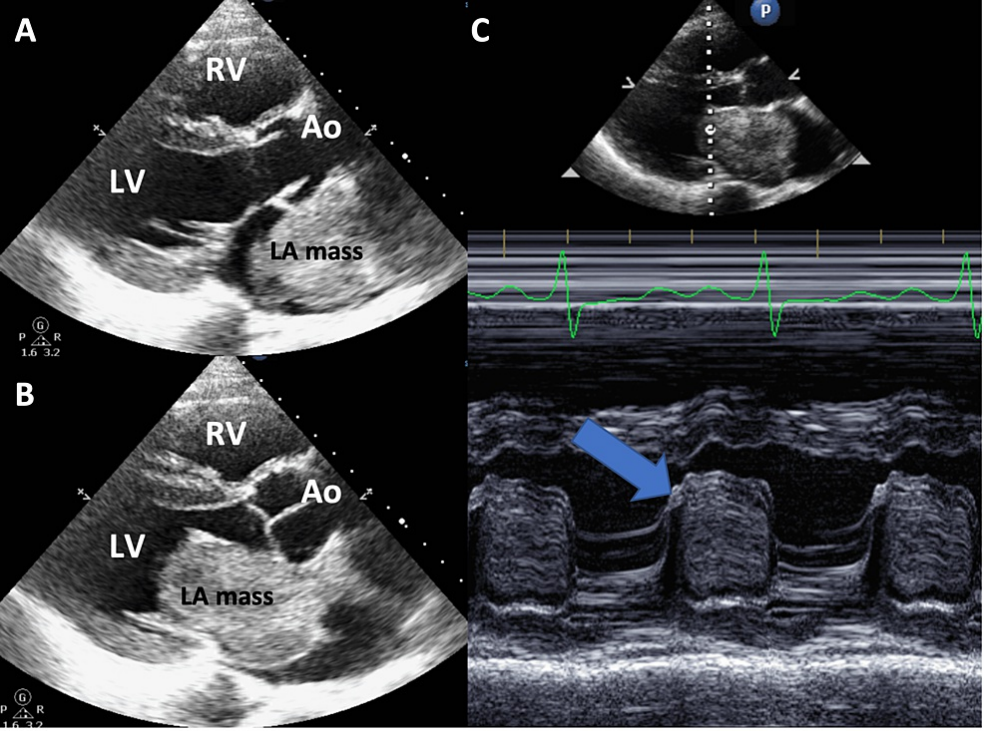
Transthoracic echocardiography in parasternal long axis view. Image A shows the large left atrial mass in LV systole. Image B shows the mass in the LV diastole protruding into the left ventricle, attached to the interatrial septum, with nearly complete obstruction of the mitral orifice. Image C is an M-mode view through the mitral leaflet showing the mass almost completely occluding the mitral orifice during LV diastole (arrow). LA, left atrium; LV, left ventricle; RV, right ventricle; Ao, aorta.
